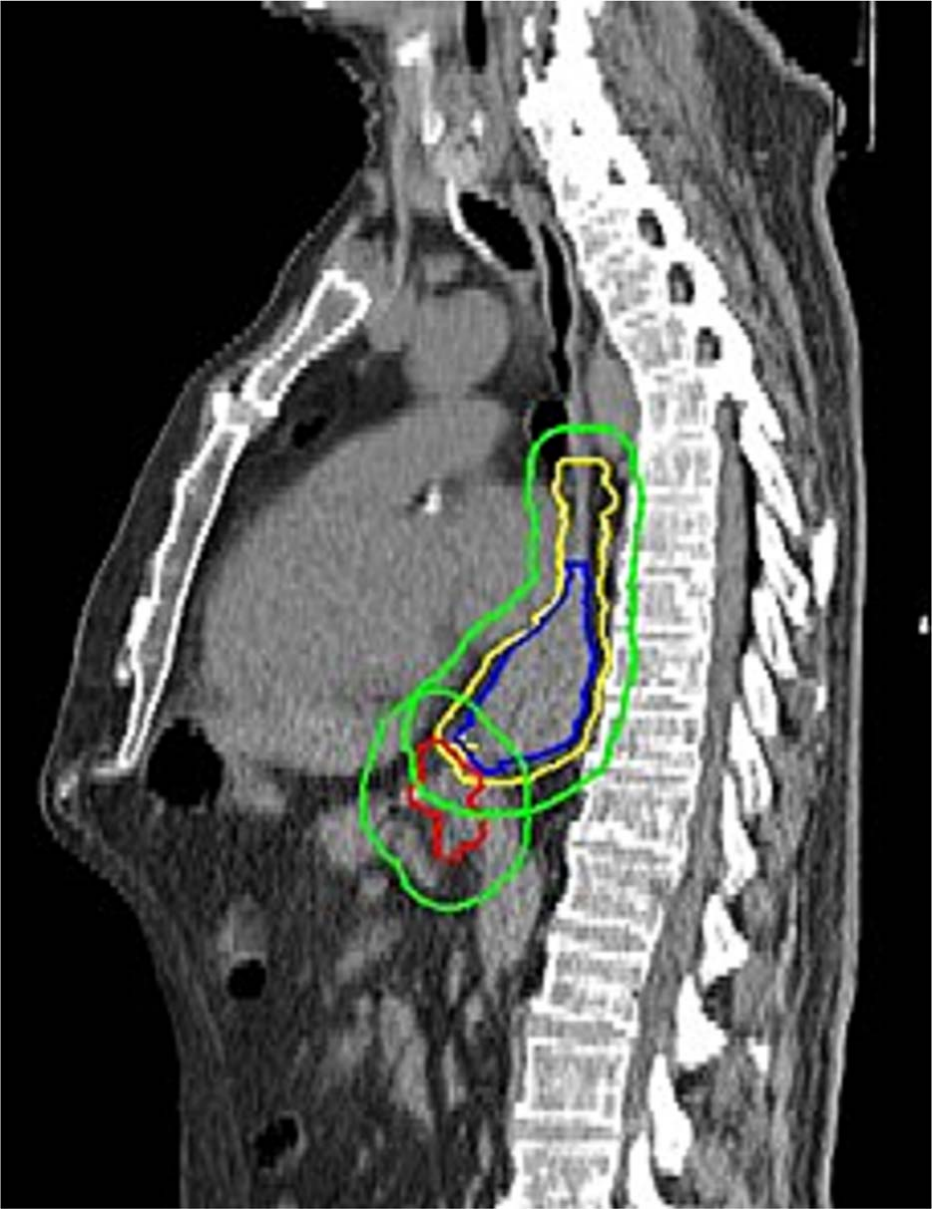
Sagittal slice of a CT scan with an example of a radiotherapy treatment plan involving delineation of the primary tumor (blue = CTV, yellow = GTV, and green = PTV) and separately metastatic abdominal lymph nodes (red).
